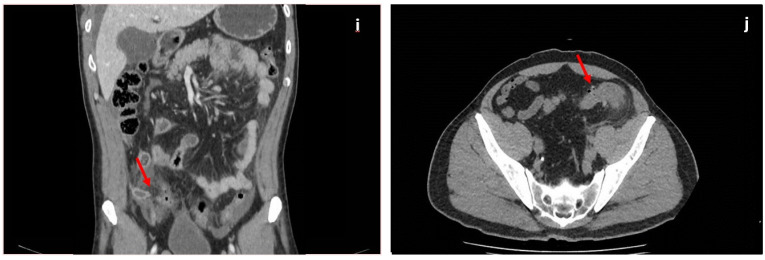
Different CT findings in abdominopelvic pathologies. Lesions are indicated by red arrows. (a) Image showing a large liver lesion; (b) image showing fat stranding and gallbladder wall discontinuity; (c) image showing a large pancreatic pseudocyst; (d) image showing left-kidney hypoattenuation during the venous phase, which is suggestive of ischemic changes; (e) image showing multiple distended small-bowel loops; (f) image showing a large left-retroperitoneal mass; (g) image showing a pneumoperitoneum; (h) aerobilia; (i) acute appendicitis coronal (fat stranding around the caecum and an inflamed appendix); (j) image showing perisigmoidian fat stranding and air pockets in acute diverticulitis.
